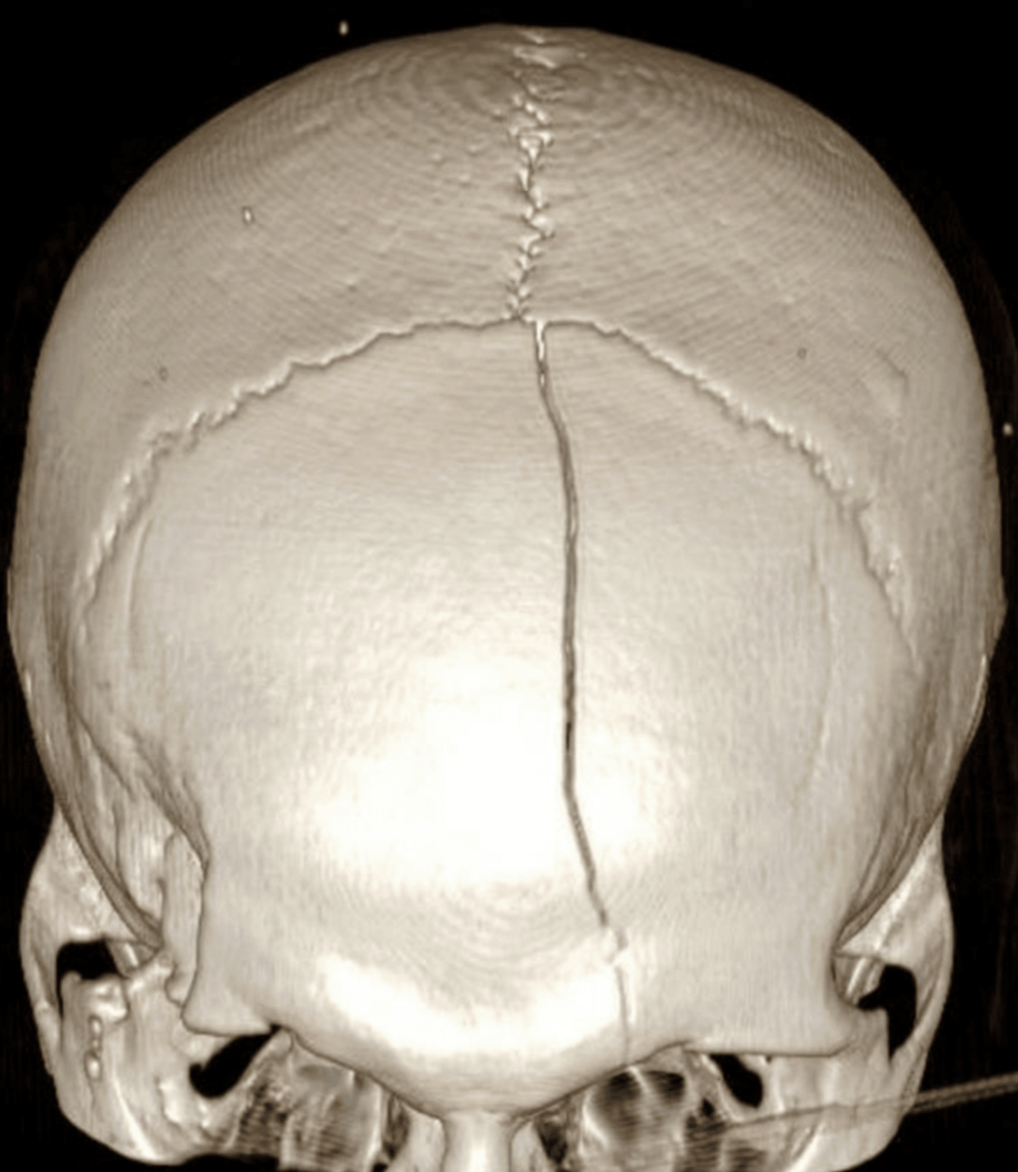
3D reconstruction of case 2 trauma CT head showing large linear frontal bone fracture 3D: three dimensional; CT: computed tomography
